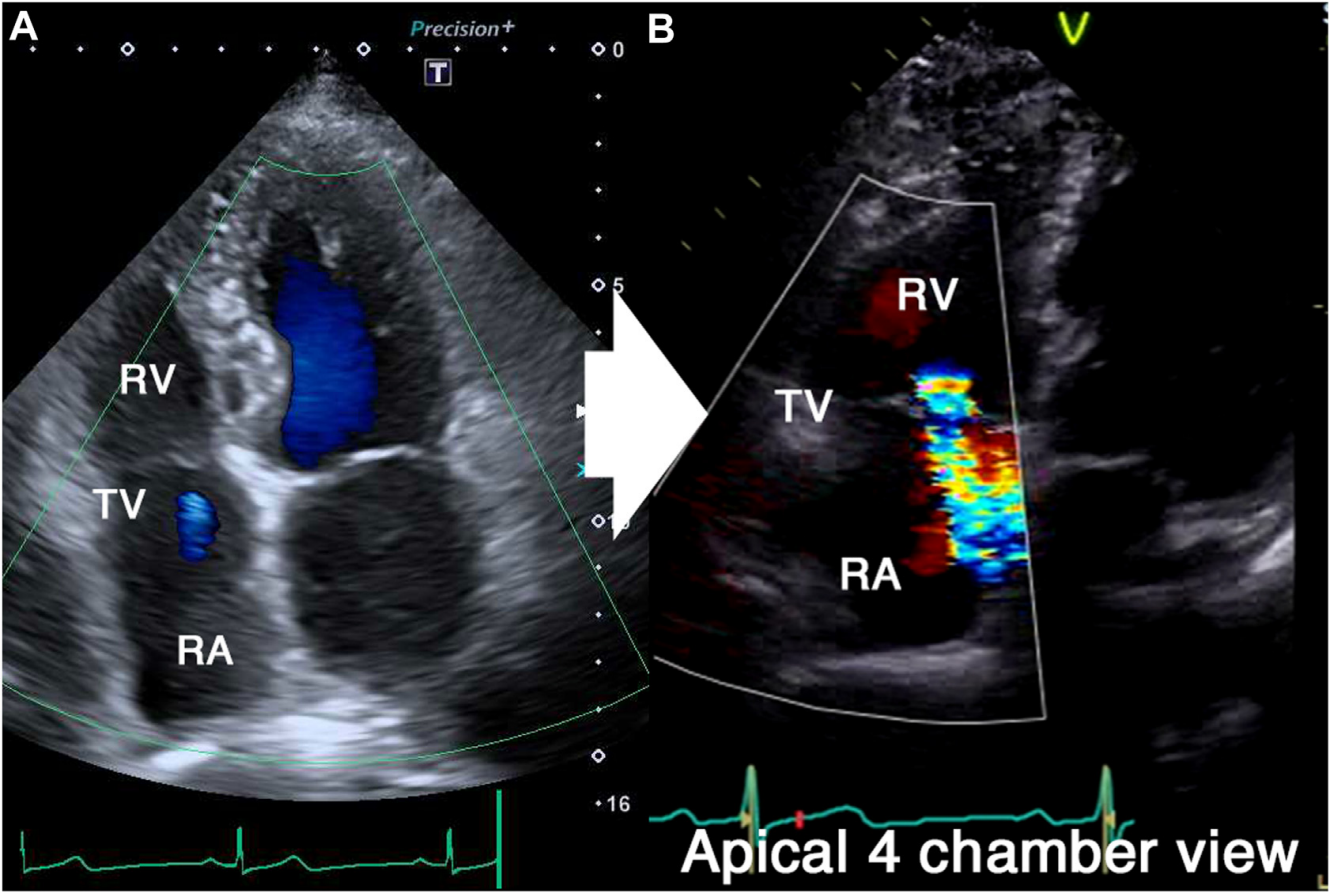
Transthoracic echocardiography before (A) and after the procedure (B). The tricuspid regurgitation worsened from trivial (A) to moderate (B). RA = right atrium; RV = right ventricle; TV = tricuspid valve.
